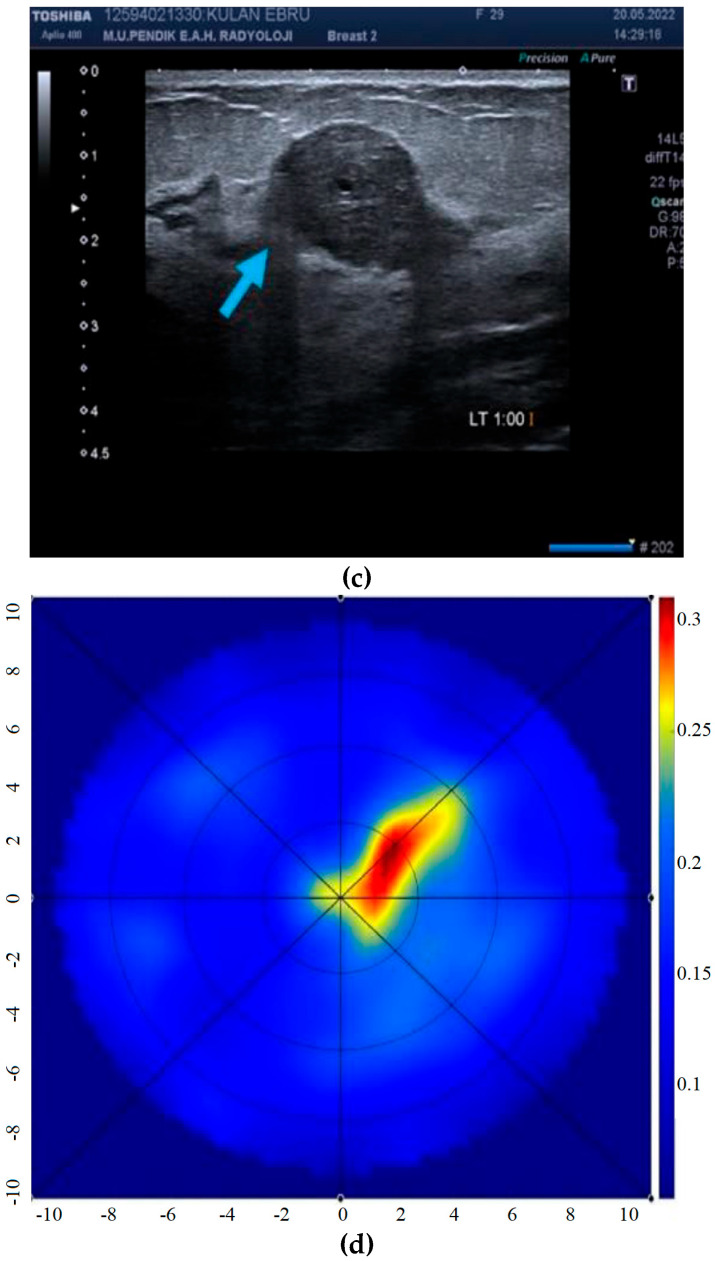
Comparison of clinical images [6]. (a) MRI image of the malignant lesion (blue circle) in a non-dense breast; (b) microwave image of the malignant lesion (high-contrast region) inside the scanning domain (blue circle) at the same location as reported via MRI; (c) ultrasound image of the benign lesion in the breast of unknown density (blue arrow); (d) microwave image of the benign lesion (high-contrast region), inside the scanning domain (blue circle), at the same location as reported via ultrasound. Images from articles published under an open-access Creative Common CC BY license.
